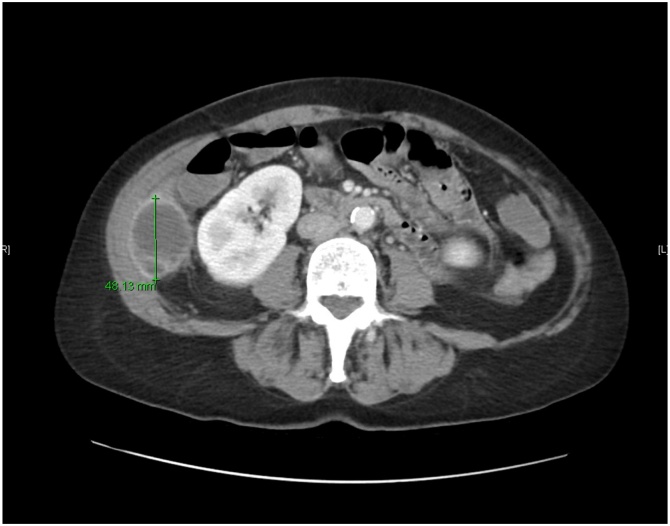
The CT of the abdomen and pelvis with contrast showed a 12.4 × 4.8 × 3.6 cm fluid collection within the right lateral abdominal wall with 2 radiopaque structures resembling gallstones.
